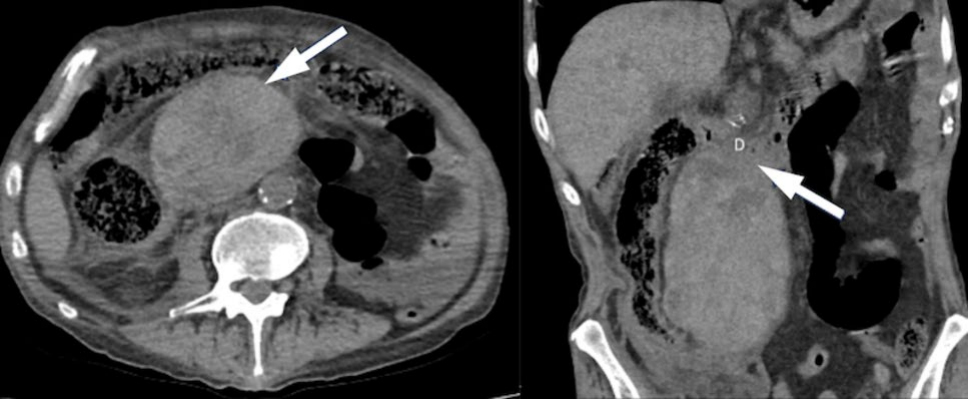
Non-enhanced CT images of an 87-year-old female patient, demonstrating an intramural duodenal hematoma affecting the second (D2) and third (D3) segments of the duodenum (arrows)
